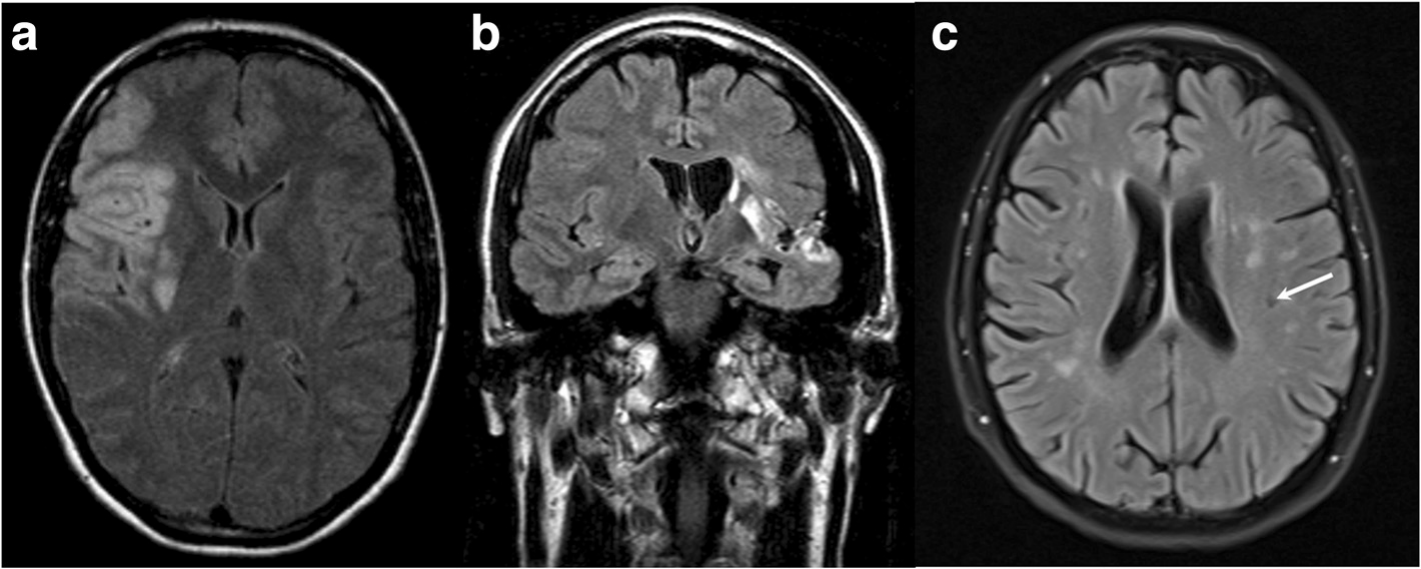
Magnetic resonance (MR) images of p.A143T patients with cerebrovascular events. a Axial MRI of the index female patient (30 years of age) suffering from an embolic stroke within the vascular territory of the right middle cerebral artery. b Coronal FLAIR-MRI of a male patient (38 years of age) suffering from an embolic stroke within the vascular territory of the left middle cerebral artery. c FLAIR-MR of a female patient (46 years of age) with TIAs and periventricular and subcortical microangiopathic and lacunar ischemic lesions (white arrow). FLAIR: fluid attenuated inversion recovery. TIA: transient ischemic attack
